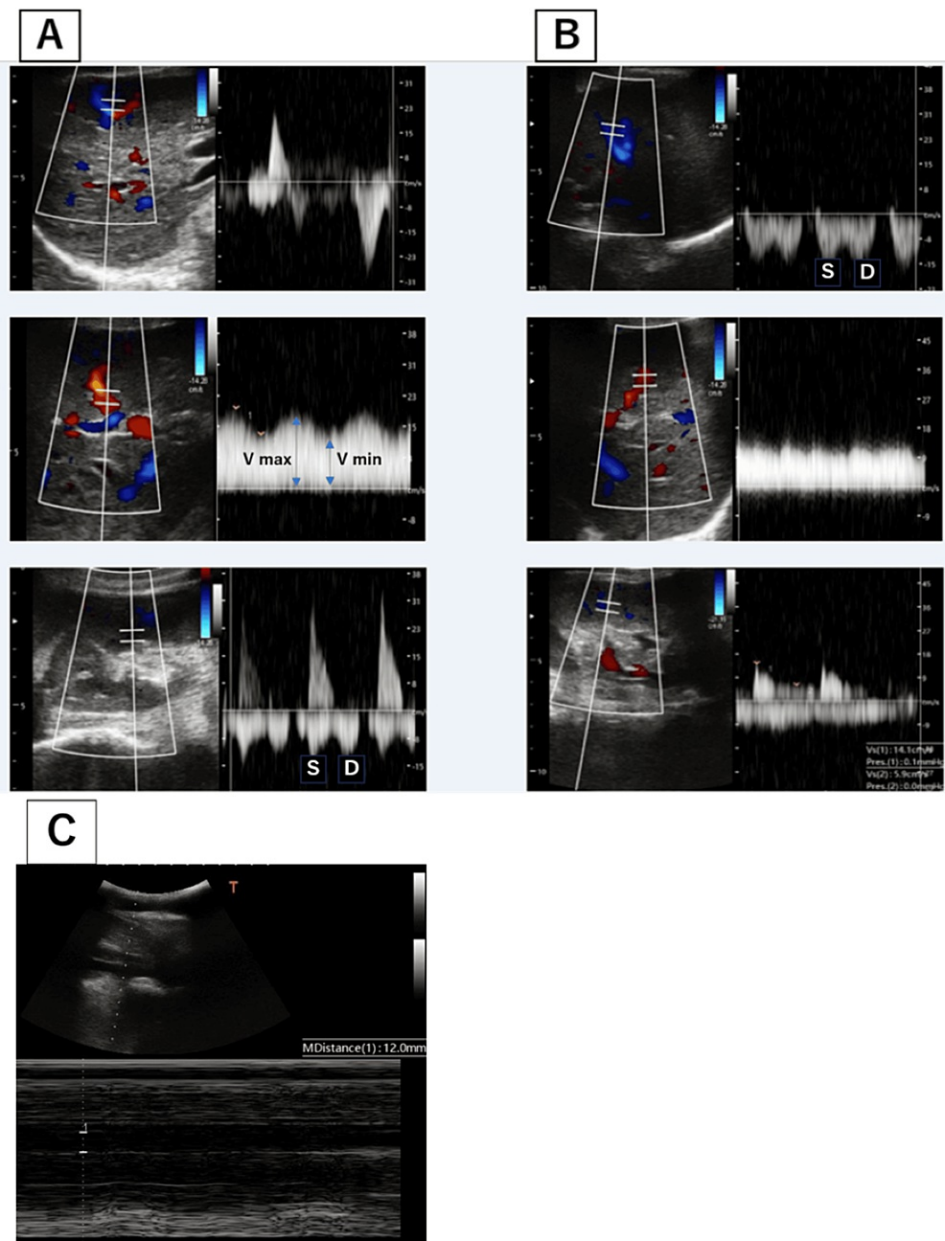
VExUS score before and after alkali administration. (A) Before alkali administration. (B) After alkali administration. (C) IVC. VExUS: Grade 2, mildly abnormal. VExUS: Grade 1, normal. VExUS = venous excess ultrasound; IVC = inferior vena cava
